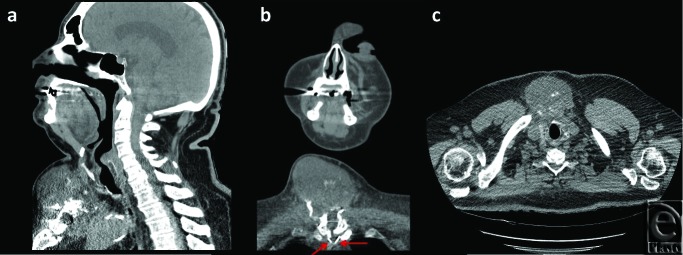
Computed tomographic scan of the soft tissue in sagittal (*a*), frontal (*b*), and transverse (*c*) planes of the granulation arising from the upper sternum. Sternal wires can be seen (*red arrows*) on the frontal view. The granulation tissue is seen extending anteriorly and posteriorly on the sagittal and transverse planes.
